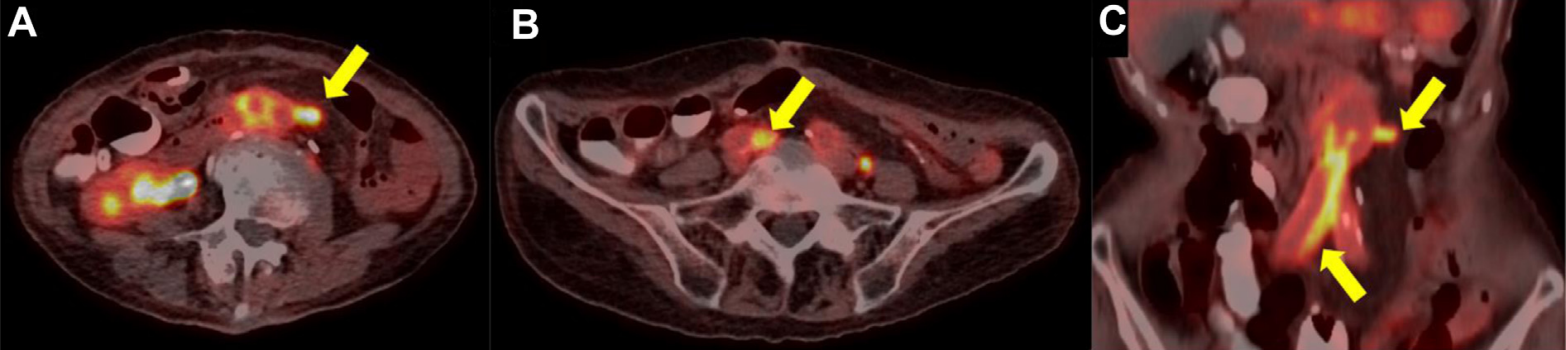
A-C, Preoperative ^18^F (fluorine-18)-fluorodeoxyglucose (FDG) positron emission tomography/computed tomography (PET/CT) scans showing abnormal ^18^F-FDG uptake (*yellow arrows*).
